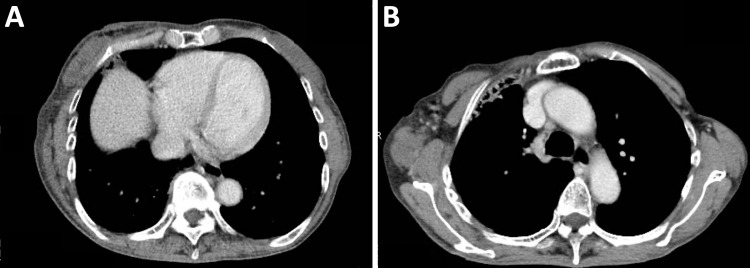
Contrast-enhanced computed tomography image obtained at four months post-treatment. (A) Primary tumor site. (B) Metastatic lymph node site.
